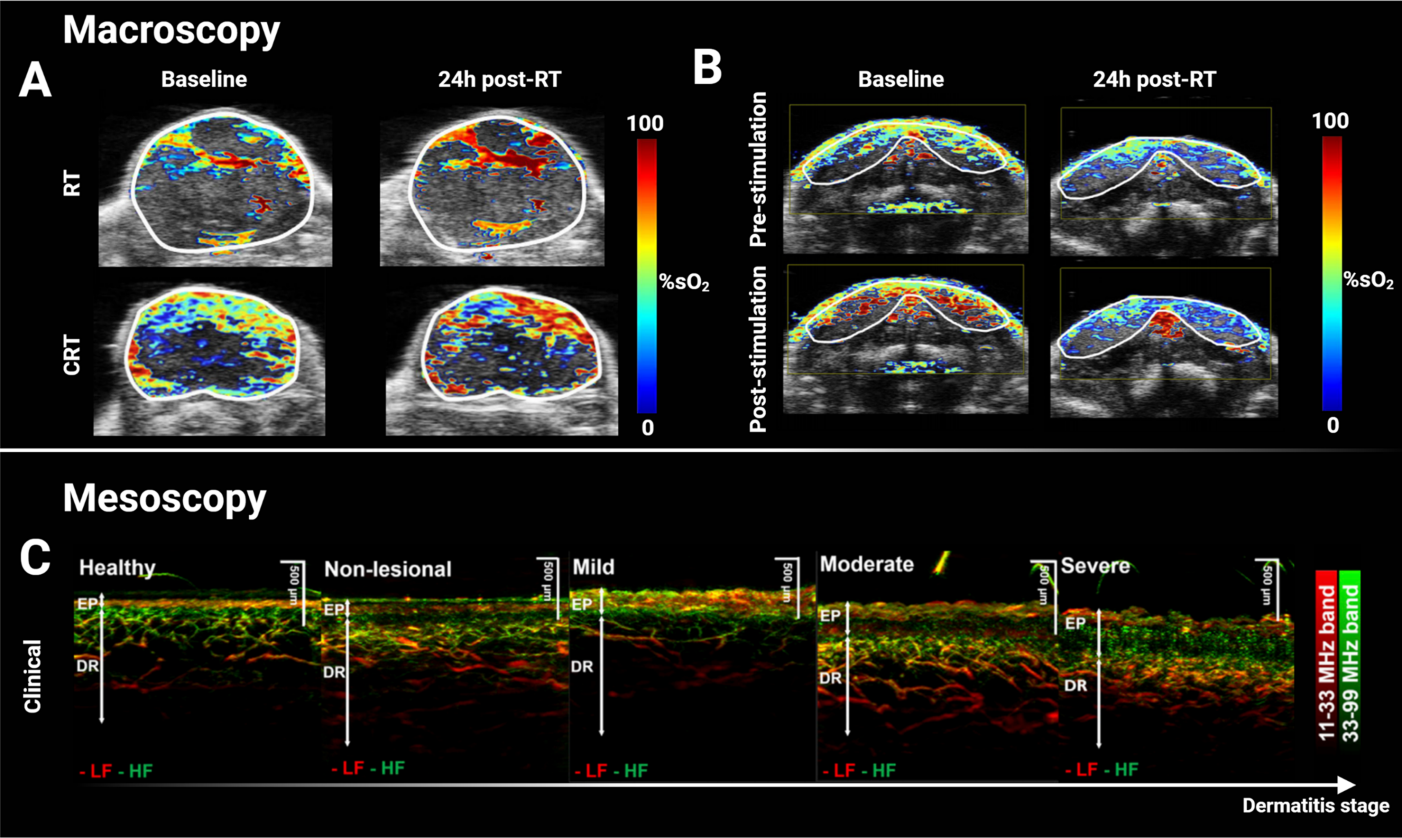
Macroscopic and mesoscopic photoacoustic imaging can monitor treatment-induced vascular changes and disease stage. (A) Multispectral optoacoustic tomography (MSOT)-derived quantitative blood oxygen saturation map (sO_2_) overlaid on co-registered ultrasound axial slice of a head & neck patient-derived xenograft tumor before and after a single dose of 15 Gy (top panel), and before and after combined 7.5 Gy of radiotherapy and administration of chemotherapeutic cetuximab. Increased sO_2_ 24h after treatment was associated with decreased tumor volume two weeks later. (B) Hemodynamic stimulation challenge of salivary glands before and after a single dose of 15 Gy with decreased change in sO_2_ response post-radiotherapy suggesting radiation-induced damage. (C) Clinical XZ maximal intensity projection of mesoscopic PAI of graded atopic dermatitis in human skin. Vascular and structural scoring could accurately grade dermatitis and such score could potentially be translated for grading radiation-induced toxicity in RT. Panels (A, B) adapted from Rich et al. (60), and panel (C) adapted from Yew et al. (61). EP, Epidermis; DR, Dermis; LF, low frequency; HF, high frequency; RT, radiotherapy; %sO_2_, percent blood oxygen saturation; CRT, chemoradiotherapy.
